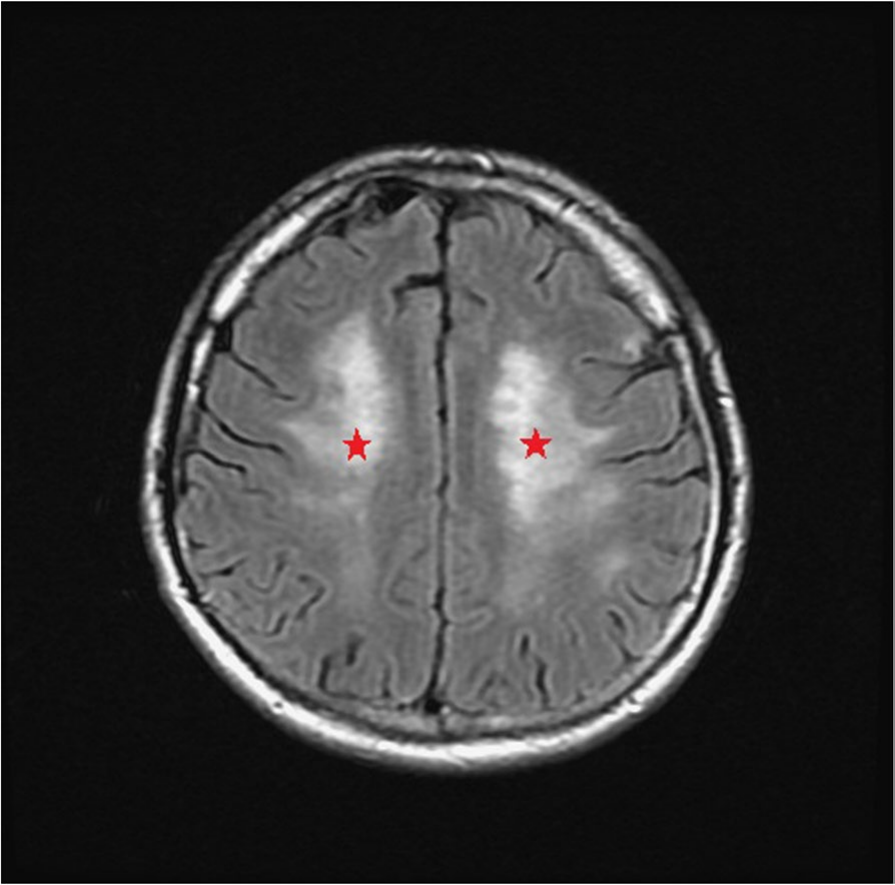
Multiple patches of high signal intensities involving bilateral periventricular white matter on T2-weighted and FLAIR image (Red stars.)
